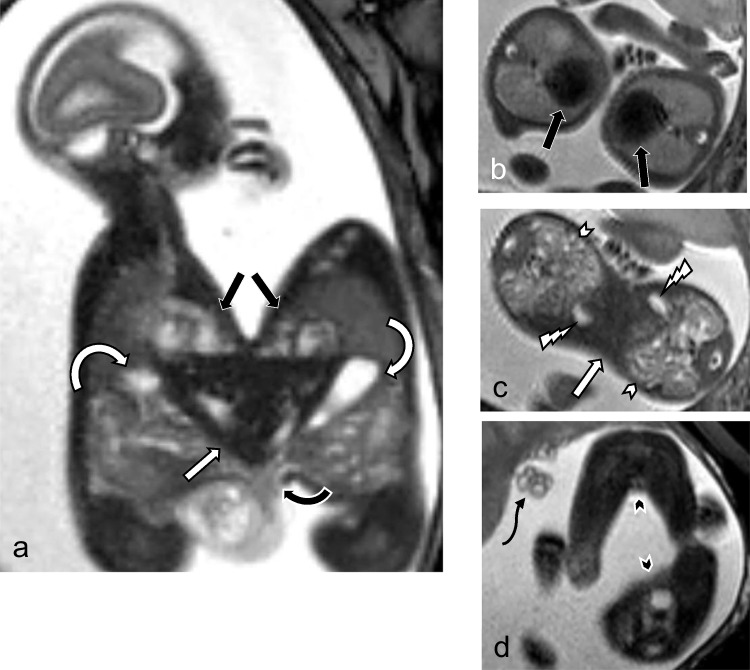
Fetal MRI of omphalopagus at gestational age 21 weeks, 3 days, showing ventral fusion of the lower chest and abdomen. a BTFE sagittal image shows separate hearts (*black arrows*), separate stomachs (*white curved arrows*), shared liver (*white straight arrow*), and shared umbilical cord abdominal insertion (*curved black arrow*). b-c T2-weighted SSH TSE sequential axial images through the torso show separate hearts (*black arrows*), shared liver (*white arrow*), separate gallbladders (*white lightning bolts*), and separation of the bowel (*white arrowheads*). d BTFE axial image shows separate pelvises (*black arrowheads*). Multivessel umbilical cord also seen (*thin curvy black arrow*). *BTFE*, balanced turbo-field echo; *SSH TSE*, single-shot turbo spin-echo
